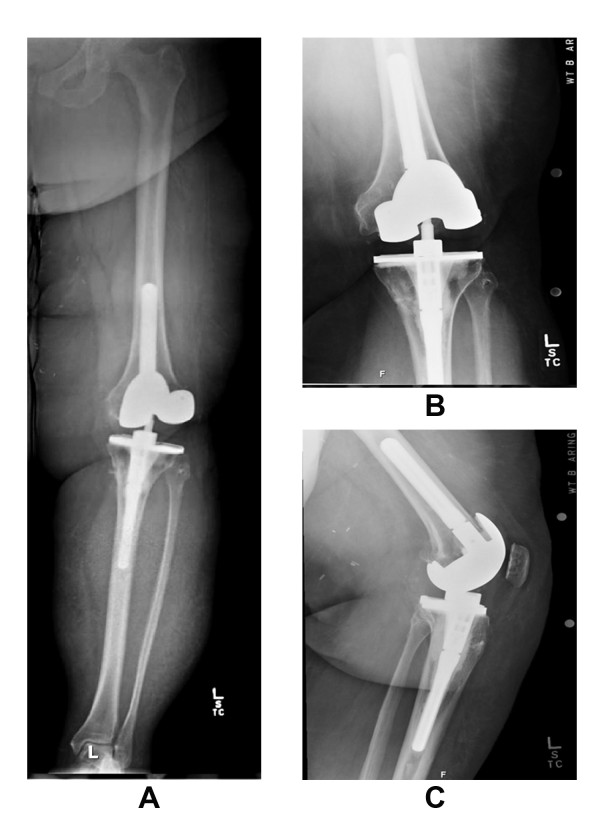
Pre-operative radiographs long standing anteroposterior (A) anteroposterior (B) and lateral view (C) of the left knee of our patient, showing significantly increased gap on the lateral side. There was no radiographic evidence of implant loosening. This lateral instability was secondary to the ligamentous failure.
